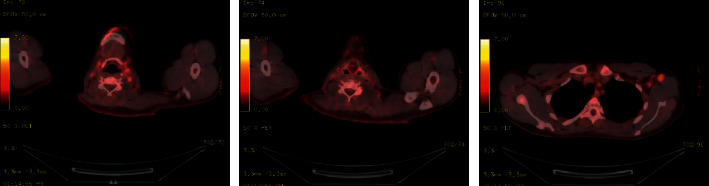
Second post treatment fused PET/CT demonstrating some FDG avidity in bilateral level 2 nodes and left axillary nodes which are reactive. The treated right jugulo-digastric mass not active or enlarged as demonstrated in Figure 1.
